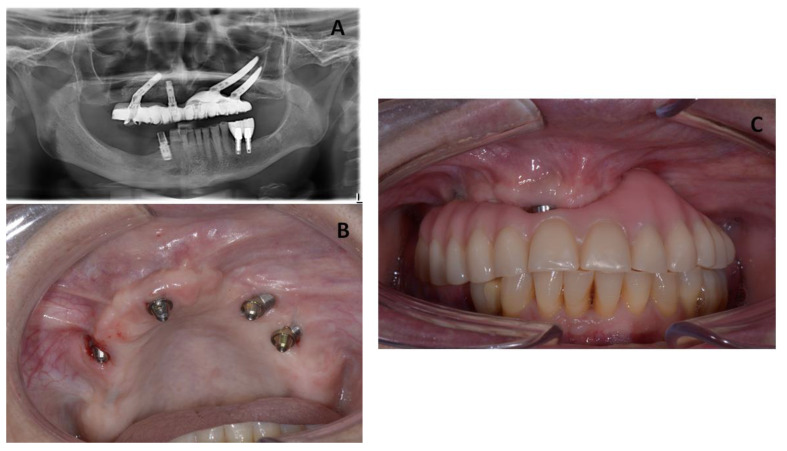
(A) Radiographic images from the patient showing healing of the MRONJ lesions with two zygomatic implants inserted after one month of follow-up. (B) Clinical images from the patient showing healing of the MRONJ lesions with two zygomatic implants inserted. (C) Intra-oral view showing the final prosthesis.
